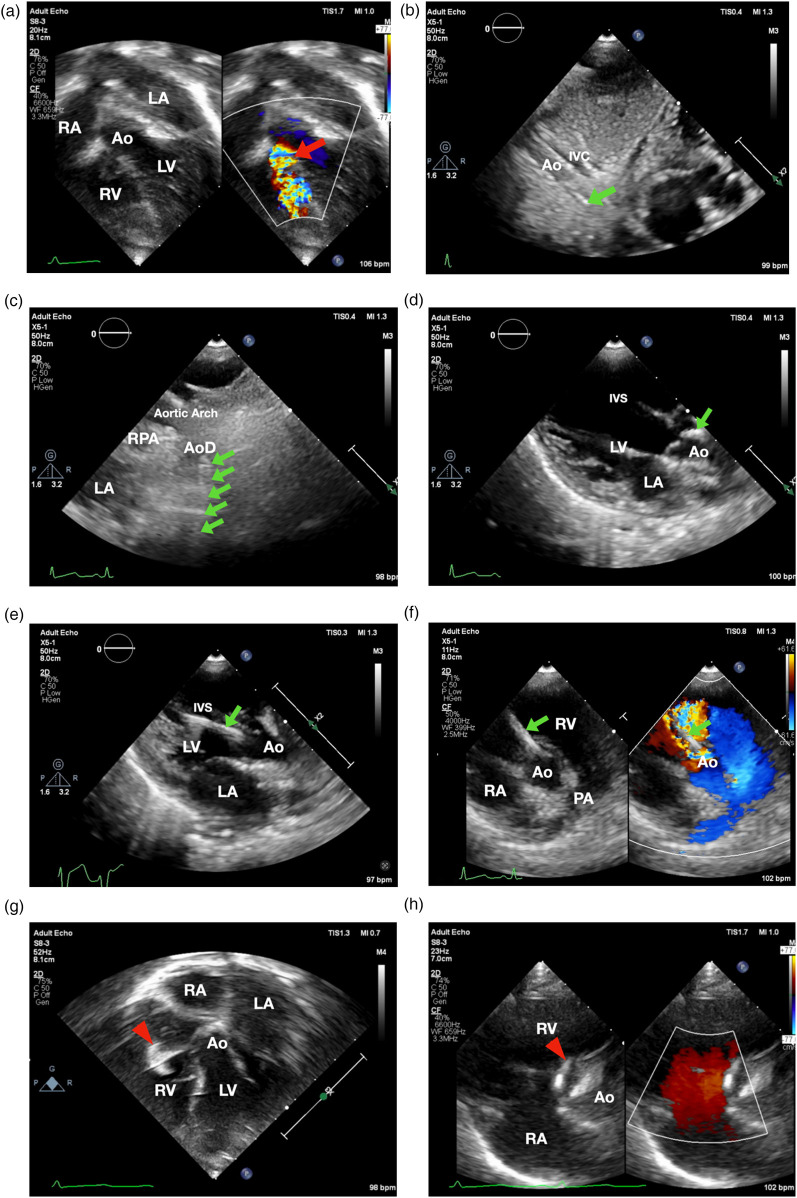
Transthoracic echocardiographynnn-guided only subaortic VSD closure in infants. (a) Apical 5-chamber view showed smallest VSD diameter 3.8 mm (showed by the red arrow). (b) 3.5/5F Guiding JR catheter with soft hydrophilic wire were entering descending aorta in subxiphoid 12 o'clock view (indicated by the green arrow) (c) suprasternal short axis view. (d) Guiding catheter was then positioned just above the aortic valve. (e) Catheter entered LV as shown in parasternal long axis view. (f) 3.5/5F Guiding JR catheter crossed the VSD in parasternal short axis view. (g) The Konar-MF VSD Occluder (Lifetech) No.7/5 mm (indicated by red arrowhead) was deployed in apical 5-chamber view. (h) Device detachment was evaluated in parasternal short axis view showing no residual shunt. Ao, aorta; AoD, descending aorta; IVC, inferior vena cava; IVS, interventricular septum; LA, left atrium; LV, left ventricle; PA, pulmonary artery; RPA, right pulmonary artery; RA, right atrium; RV, right ventricle.
